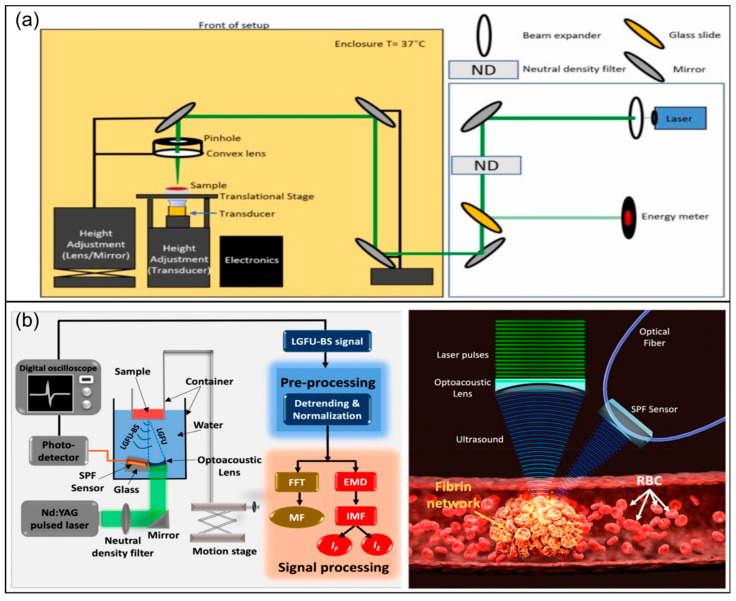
Typical mechanisms and results of optic–acoustic coupled biosensing technologies for coagulation testing. (a) Photoacoustic coagulation biosensing assay using water as medium. Figure reproduced from ref. [49]. CC BY license, Elsevier GmbH, copyright © 2023, the authors. (b) Design and mechanism of an in vivo acousto-optic biosensing method for fibrin monitoring based on all-optical ultrasound spectroscopic sensing: light and sound propagate through water, and the clot absorbs light and generates vibrations that are captured by acoustic sensors. Figure reproduced with permission from ref. [47]. Copyright © 2022, American Chemical Society.
